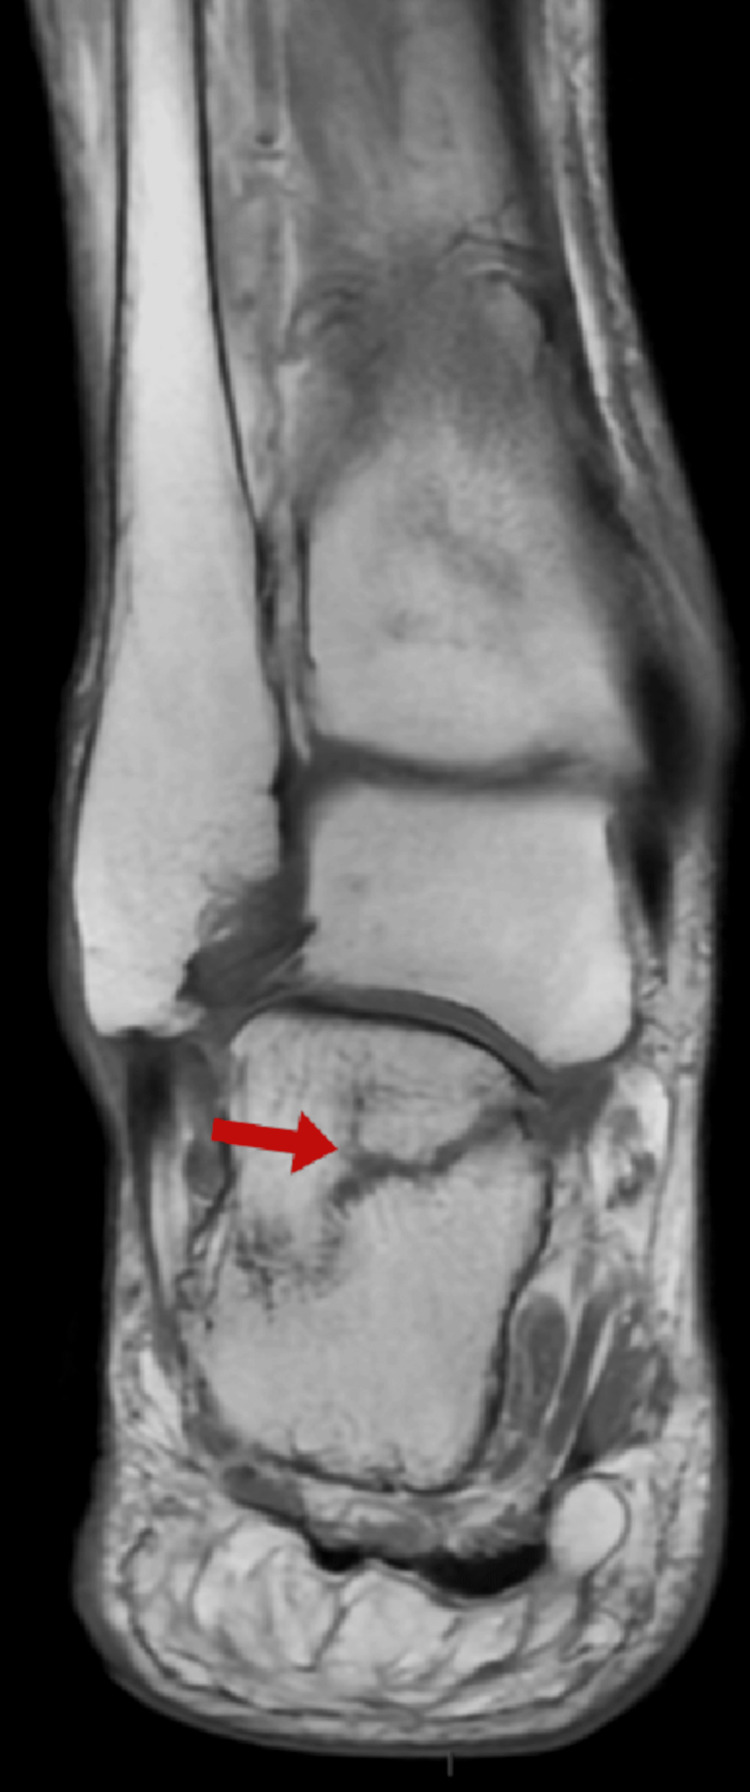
Sagittal T2-weighted MRI demonstrating fracture line of the calcaneus MRI: magnetic resonance imaging
